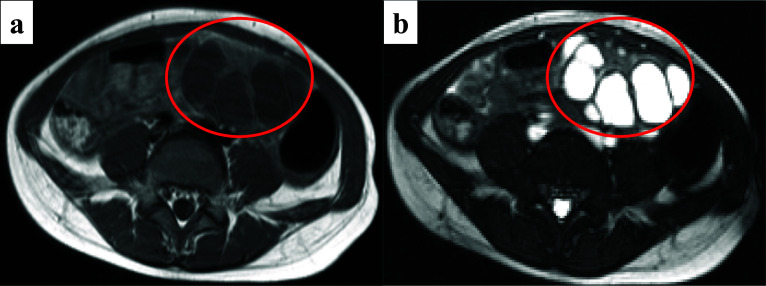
MRI findings. a Contents of the cyst showed low signal intensity on T1-weighted imaging. b Contents of the cyst showed high signal intensity on T2-weighted imaging
